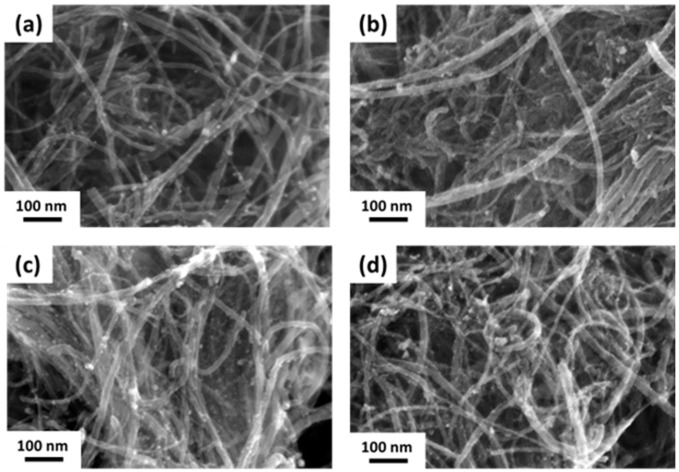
FE-SEM images of Pd/A-CNT after synchrotron X-ray irradiation for (a) 0.5, (b) 1, (c) 2, and (d) 4 min, respectively.
